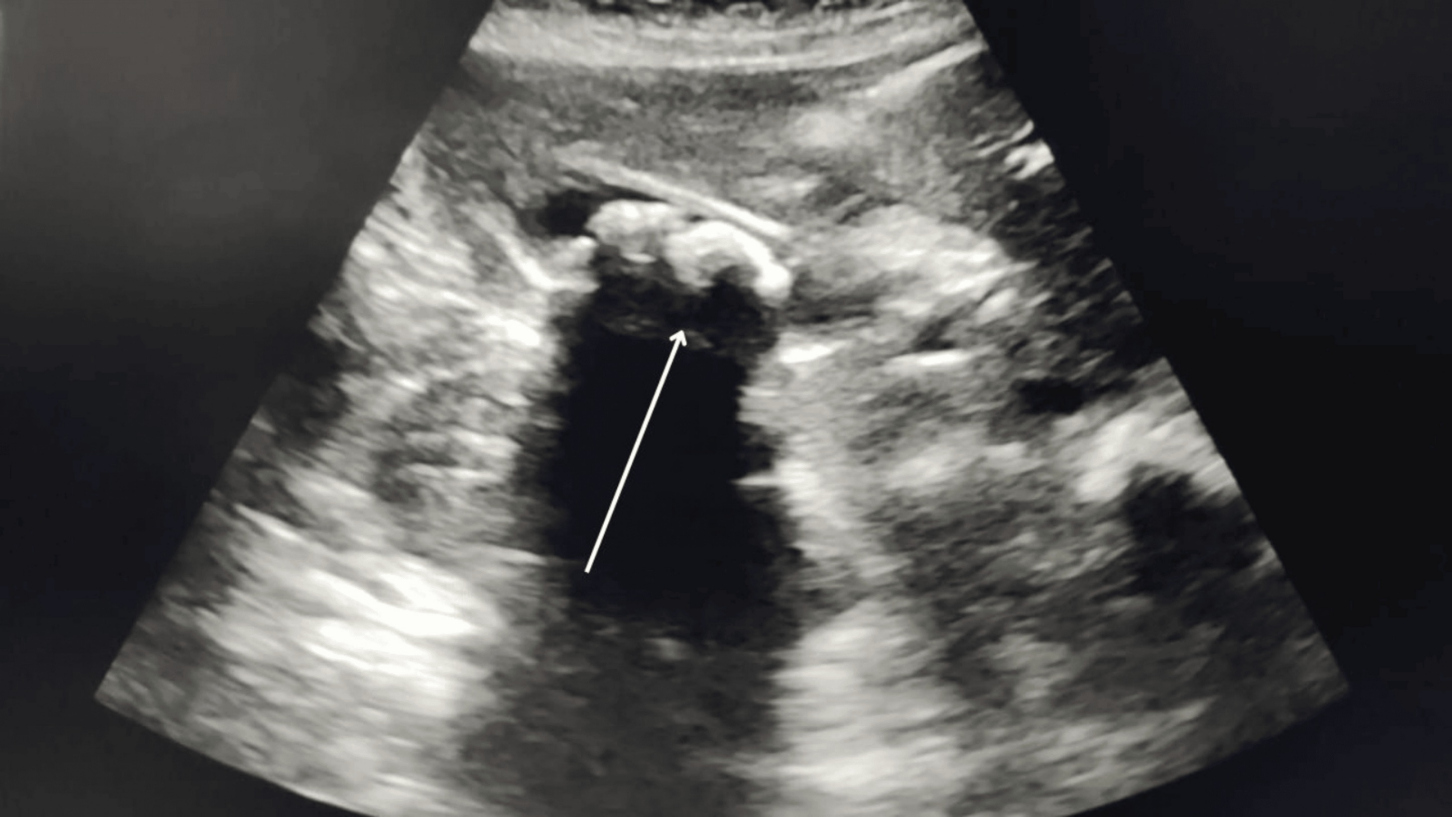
Ultrasound image showing the gallbladder with calculi (marked with a white arrow)
